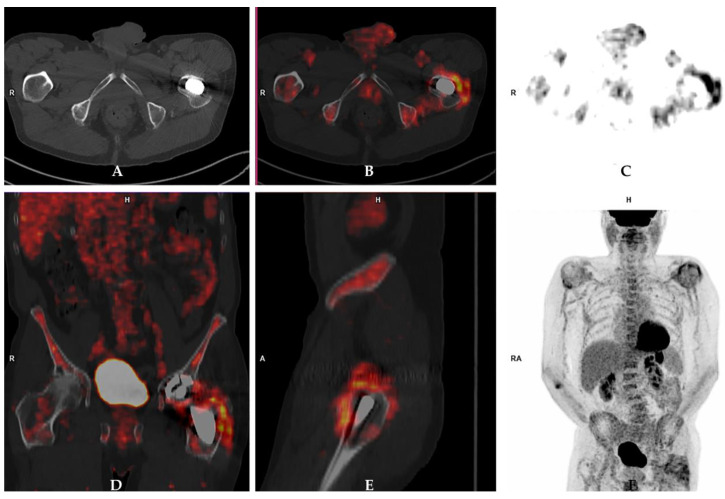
Prosthetic joint infection detected by 18Fluorine-fluorodeoxyglucose positron emission tomography/computed tomography ([^18^F]-FDG-PET/CT) in a 72-year-old male patient who underwent left hip arthroplasty four years before the PET/CT scan. [^18^F]-FDG-PET/CT images (A: axial view of CT scan; B: axail fused images; C: axial PET images; D: coronal [^18^F]-FDG-PET/CT view; E: sagittal [^18^F]-FDG-PET/CT view; F: maximum intensity projection [^18^F]-FDG-PET images) showed increased radiopharmaceutical uptake in the periprosthetic bone and soft tissues at the level of the femoral component of the prosthesis. These findings were indicative of prosthetic joint infection, which was confirmed by further examinations including microbiological culture.
